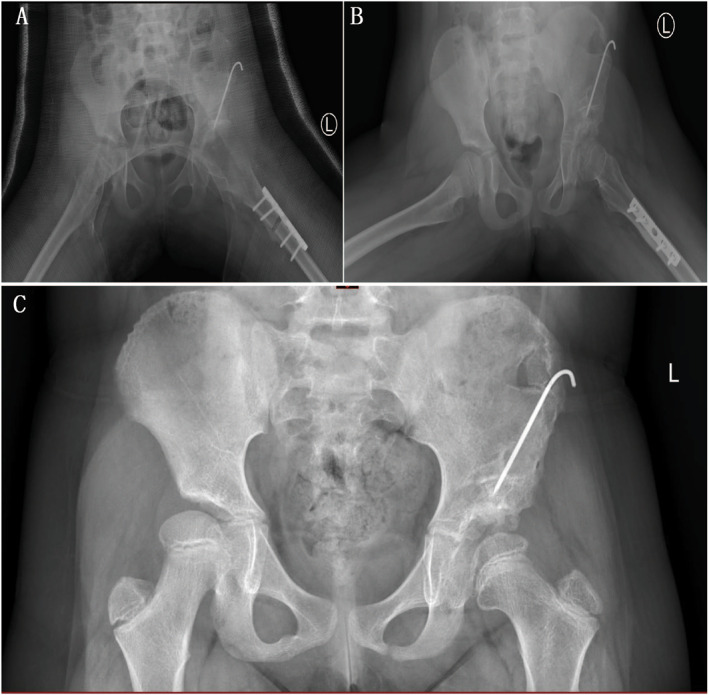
Anteroposterior radiographs of the pelvis during follow‐up after Pemberton osteotomy. Three days after surgery (A) 3 months after surgery (B), and 6 months after surgery (C).
